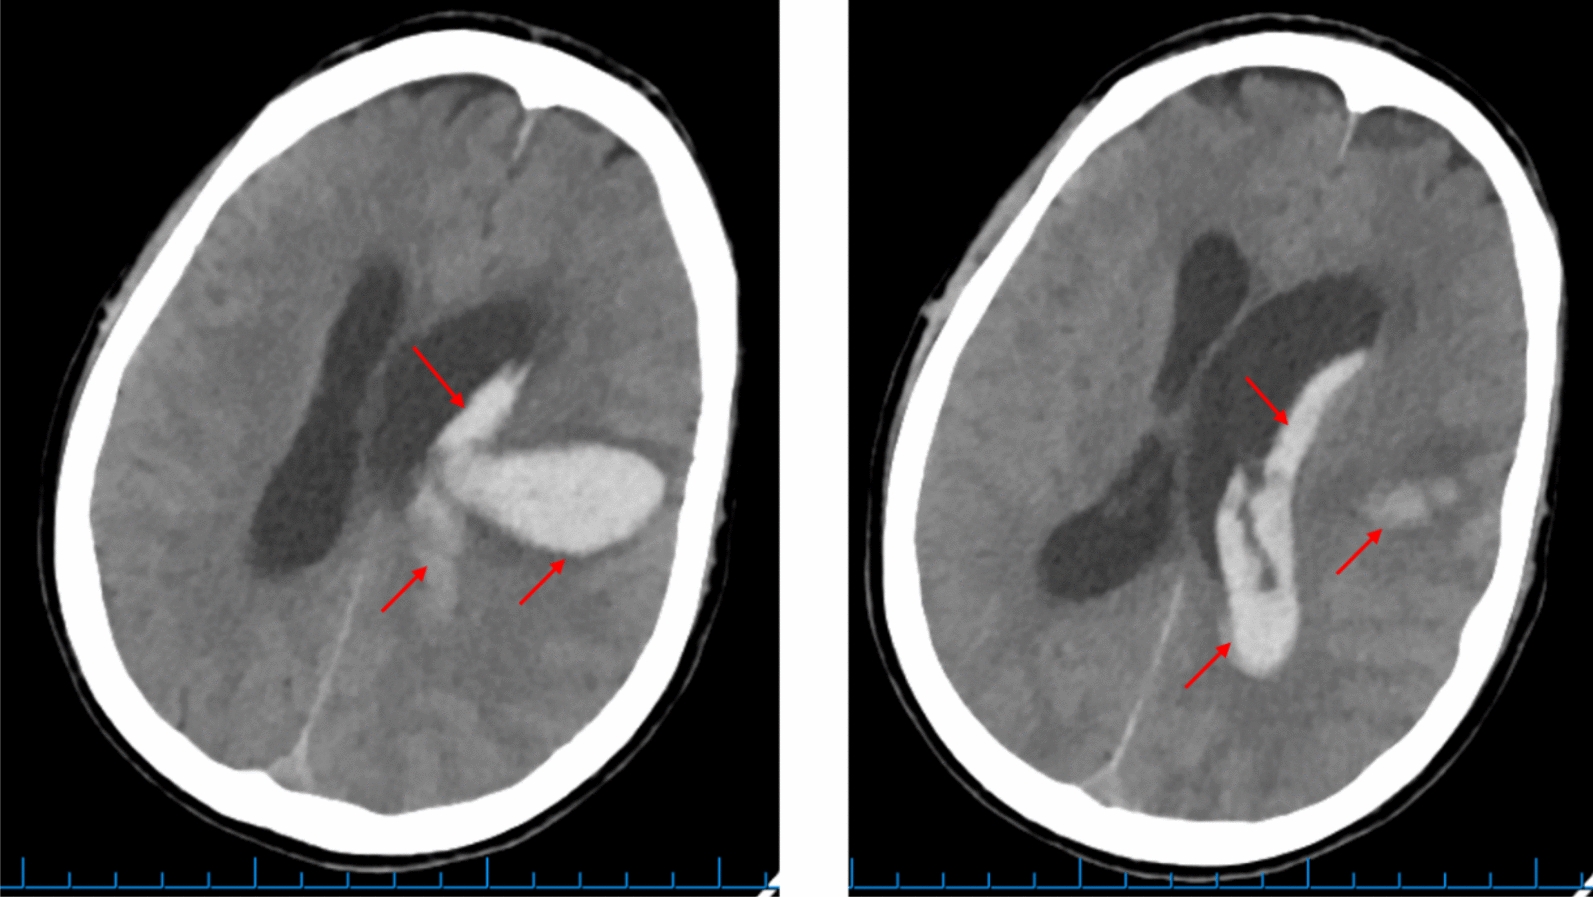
Hemorrhage on contrast-enhanced brain CT scan on day 19. Hemorrhage in the parietal area, adjacent to the left lateral ventricle (arrows), D# 2.9 × 4.3 × 4.7 cm, and left lateral intraventricular hemorrhage shifts the midline to the right, *CT scan* computed tomography scan
